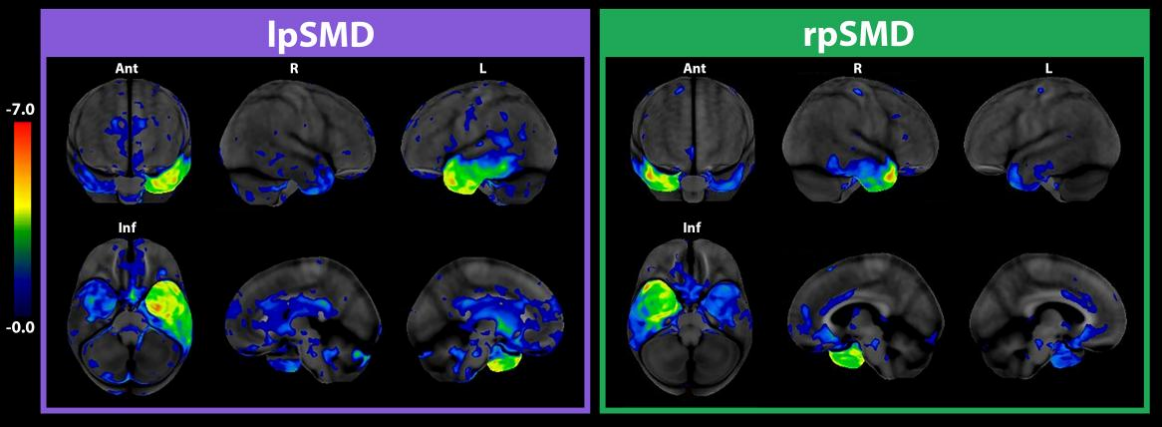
Pattern of FDG-PET hypometabolism in SMD variants. The CortexIDs show bilateral yet markedly asymmetric hypometabolism of the anterolateral and medial temporal lobes. The hypometabolism is left lateralized in lpSMD and right lateralized in rpSMD. The pattern of hypometabolism in the predominantly involved hemisphere is reminiscent of a ‘boxing glove’. Ant, anterior; Inf, inferior; L, left; lpSMD, left-predominant semantic dementia; R, right; rpSMD, right-predominant semantic dementia.
